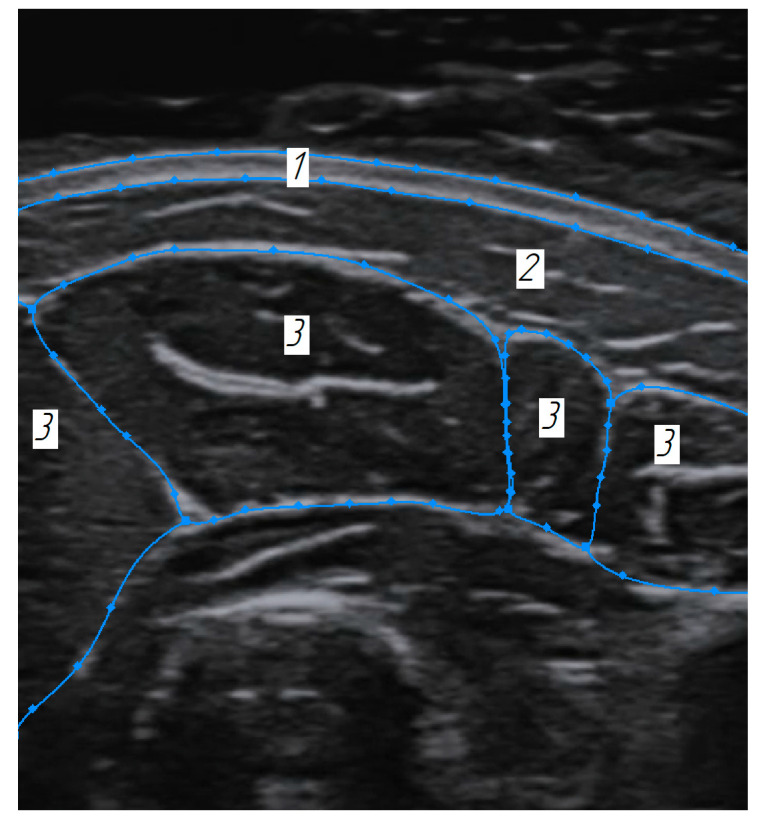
Sonogram of the cross-section of the forearm: 1—skin layer, 2—subcutaneous fat layer, 3—superficial muscles’ boundaries.
